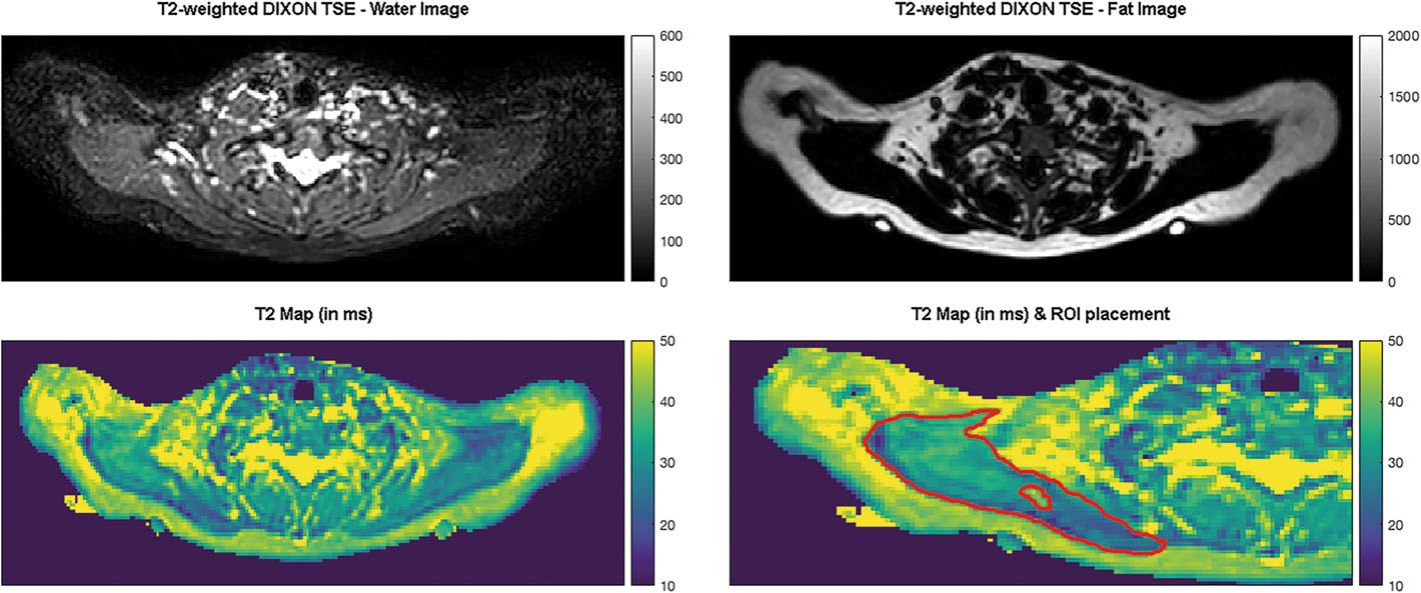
Magnetic resonance imaging (MRI) including T2 mapping of the upper trapezius muscles. This figure captures an exemplary case by showing representative axial slices of the T2-weighted DIXON turbo spin echo (TSE) sequence (upper row). The left upper corner shows the T2-weighted DIXON TSE water image, the right upper corner captures the T2-weighted DIXON TSE fat image. Furthermore, T2 maps as derived from the T2-prepared TSE sequence are displayed (lower row). The left lower corner pictures the color-coded T2 map, the right lower corner shows the same color-coded T2 map after manual placement of regions of interest (ROIs) in the right upper trapezius muscle and with respect to a signal alteration (T2 elevation) within the muscle. In this exemplary case, the signal alteration was located in the area of a manually defined myofascial trigger point (mTrP), as indicated by the spatial relation to superficially attached nitroglycerine capsules as markers. The signal alteration in terms of the circumscribed T2 elevation shows a T2-hyperintense correlate in the T2-weighted DIXON TSE water image
